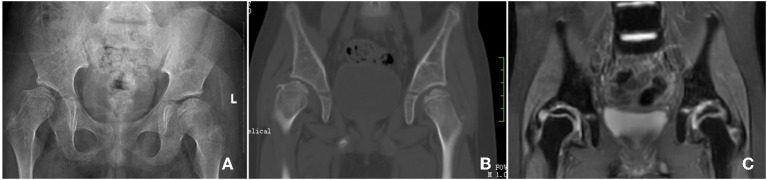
Follow up Imaging examination, X-ray shows the shape of the femoral head was remodeling (A); the follow up CT result shows the microcystic area was replaced by normal bone tissue (B); the follow up MRI result shows the multiple small patchy T2WI hyperintensity shadows under the right femoral metaphysis was disappear (C).
